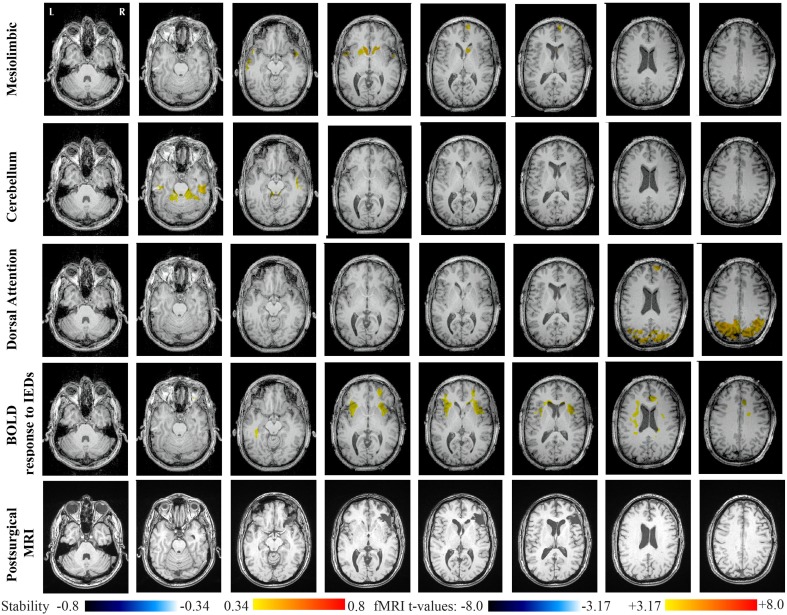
Evaluation of DANI on Patient 2 with right Orbito-Frontal epilepsy. Results are presenting most salient stability changes observed in *Cmap*_*n*_ followed by a t-map of the BOLD response to epileptic discharges and a postsurgical T1 MRI, all resampled in the native anatomical MRI space of the patient. Most salient stability changes observed for *Cmap*_*n*_ of Mesolimbic, Cerebellum, and Dorsal Attention networks are presented. The Dorsal Attention network was also involved in significant interactions of modularity changes at *p* < 0.001. Same colormap conventions than in Figure 6.
